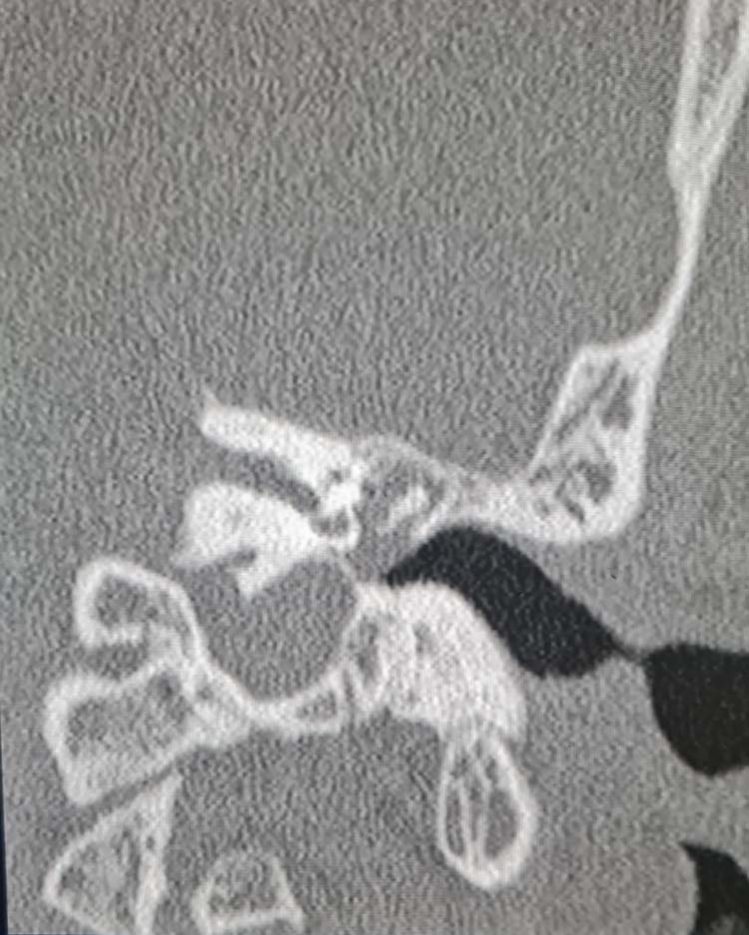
Coronal computed tomography image showing the mass with soft tissue density filling the middle ear cavity.
